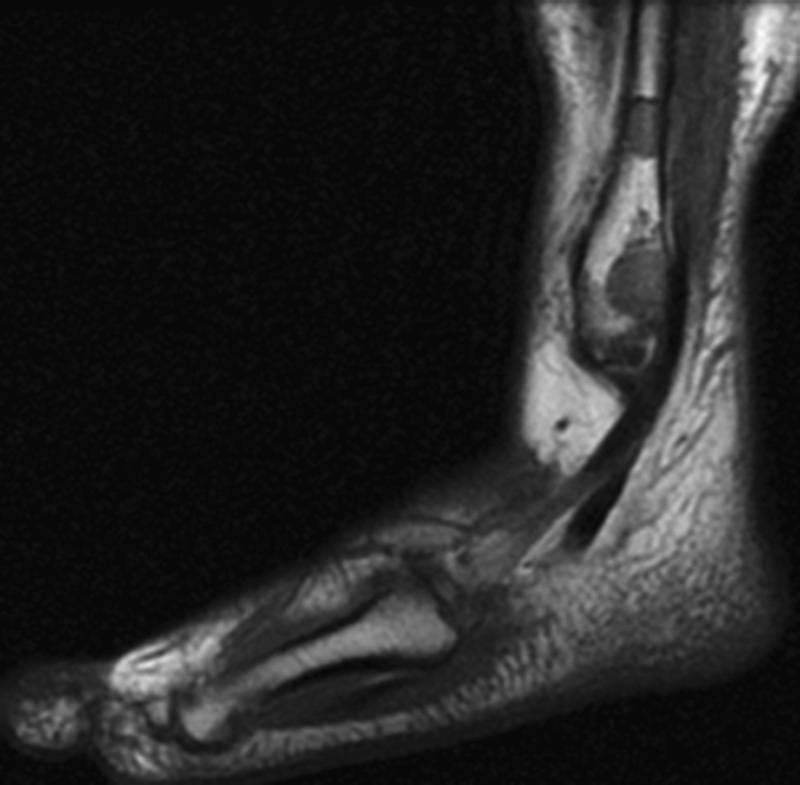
Hypointense lesions in fibula (T1-weighted magnetic resonance imaging section).
